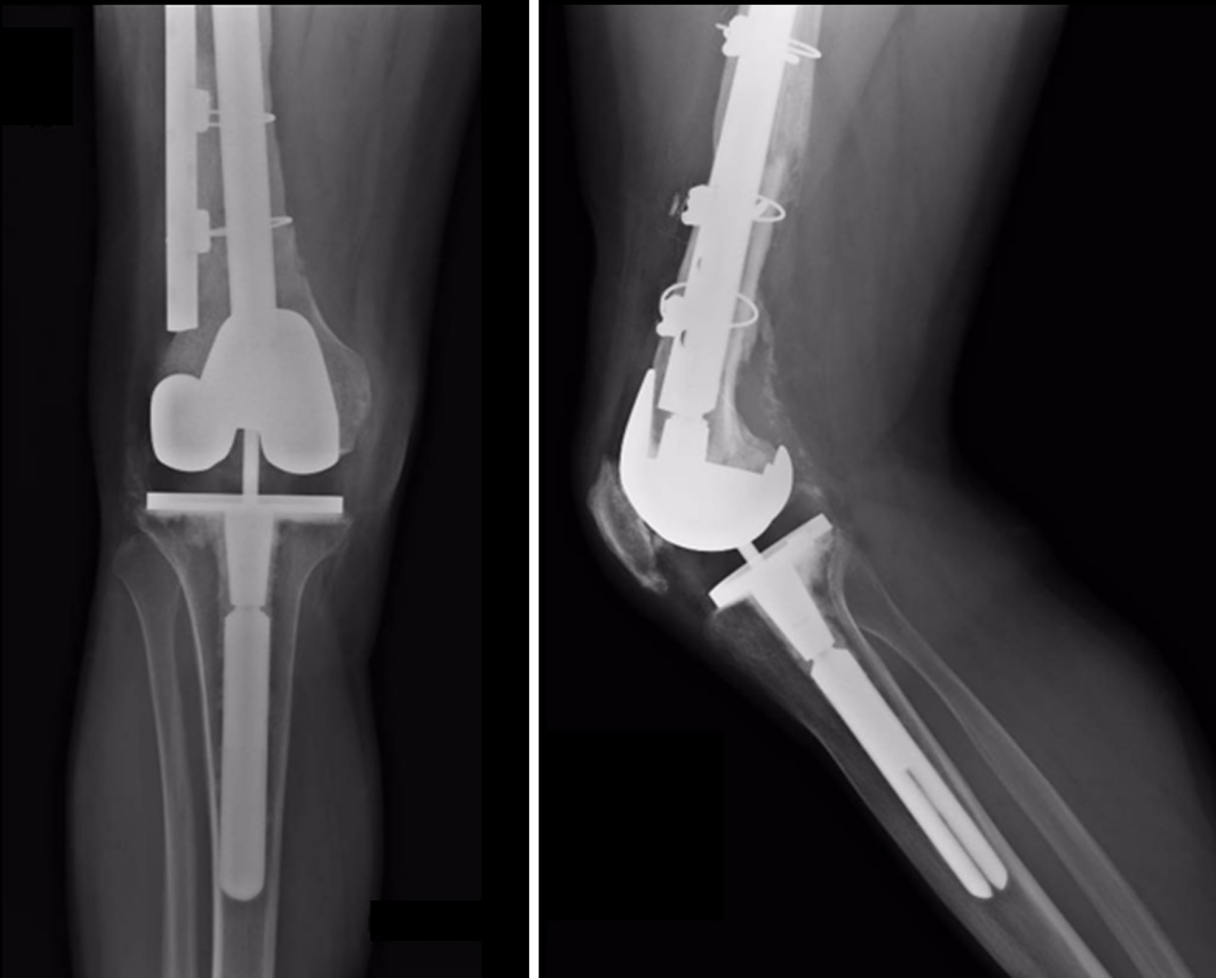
Anteroposterior and lateral knee X-rays taken 10 years after allograft revision. Revision knee implant is still stable. No problems have been recorded or reported by the patient.
